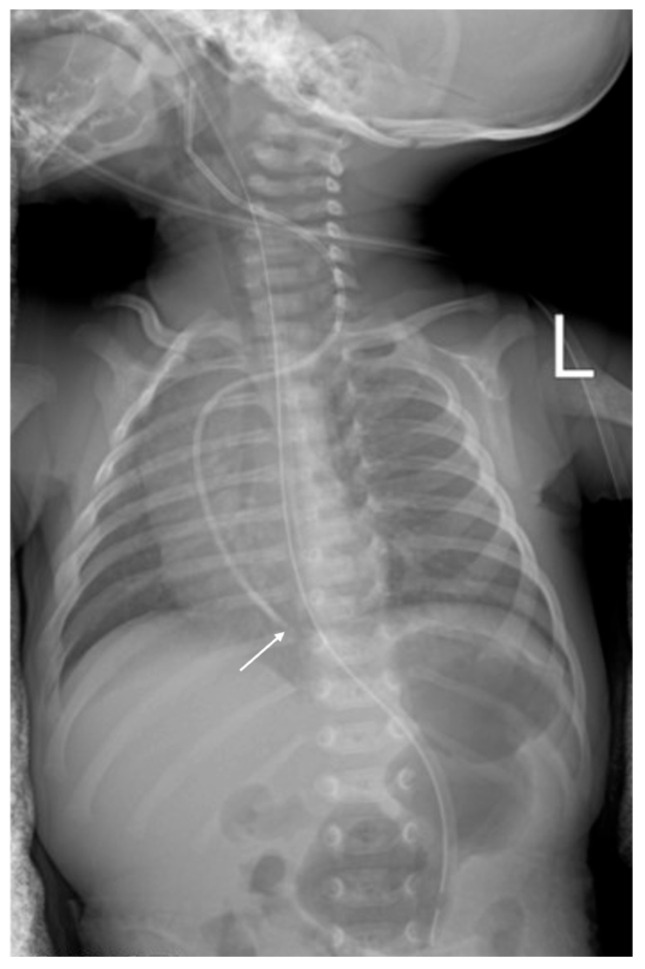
Chest radiography of Patient 1 right after CVC placement. Catheter tip located intracardially (white arrow). L: left.
